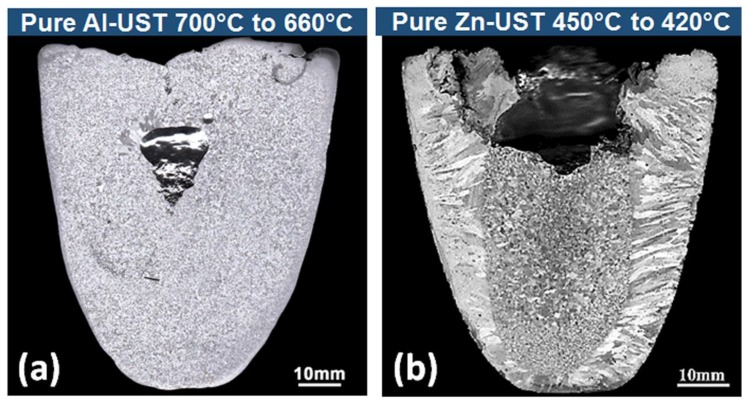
Macrostructures of ultrasonically treated (a) pure Al and (b) pure Zn.
